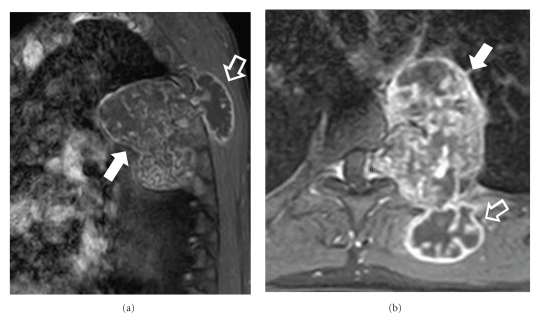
MR imaging of the same patient. The sagittal (a) and axial (b) contrast-enhanced fat-saturated T1-w MR images show intense heterogeneous enhancement of both the intrathoracic (arrows) and the superficial (open arrows) tumor components. Enhancement is also observed in the intraforaminal component of the tumor (asterisk).
